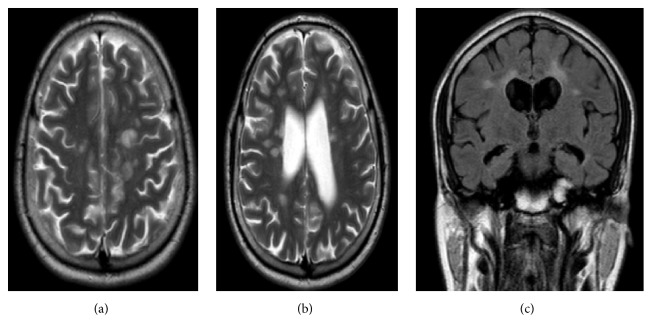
(a and b) Axial T2 MRI shows multiple periventricular and juxtacortical high intensity lesions. (c) Coronal flair MRI shows periventricular and juxtacortical high intensity lesions.
